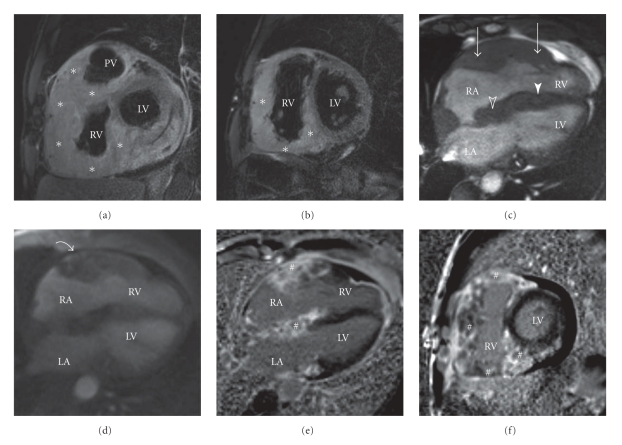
T2-weighted short inversion-time, inversion-recovery (STIR) breath hold pulse sequences ((a) and (b)) showing concentric thickening and oedema of the right ventricle. The infiltration (asterisk) extends around the root of the pulmonary artery. Four-chamber SSFP cine view (c) showing infiltration of the right ventricle (arrow), right atrium (empty arrow), interatrial septum (empty arrowhead), and basal to mid interventricular septum (arrowhead). Four-chamber first-pass T1-weighted multishot gradient-echo echo-planar sequence (d) shows partial hyperenhancement (arrow) of the right ventricle in support of an inflammatory process. Four-chamber (e) and short-axis (f) three-dimensional phase-sensitive inversion recovery sequences demonstrating widespread and heterogeneous enhancement (#) of the right atrium and ventricle. RA indicates right atrium; LA, left atrium; RV, right ventricle; LV, left ventricle; PV, pulmonary valve.
